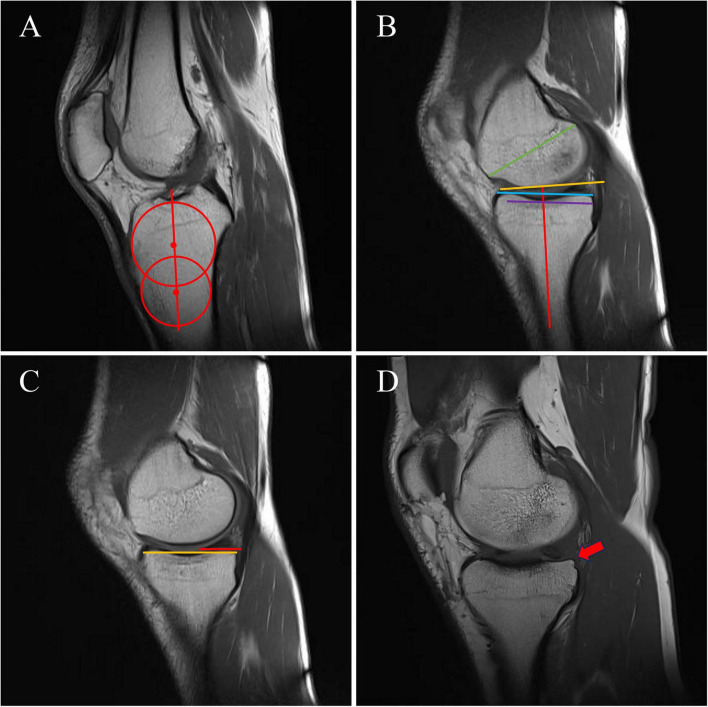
Measurement of osseous morphologic parameters on sagittal MRI. (A) Determination of the tibial anatomical axis (red line). (B) The MTS was calculated as *90*° *minus* the angle between the tibial anatomical axis and the blue line. The MTPD was defined as the perpendicular distance between the blue line and the purple line. The MMS was measured as the angle between the tibial anatomical axis and the yellow line. The MFCL was defined as the length of the green line, and the MTPL as the length of the blue line; the ratio MFCL/MTPL was then computed. (C) MMPHr was calculated as the ratio of the red-line length to the yellow-line length. (D) The red arrow indicates a posterior tibial osteophyte. MTS, medial tibial slope; MTPD, medial tibial plateau depth; MMS, medial meniscal slope; MFCL, medial femoral condyle length; MTPL, medial tibial plateau length; MMPHr, medial meniscus posterior root tibial coverage ratio.
